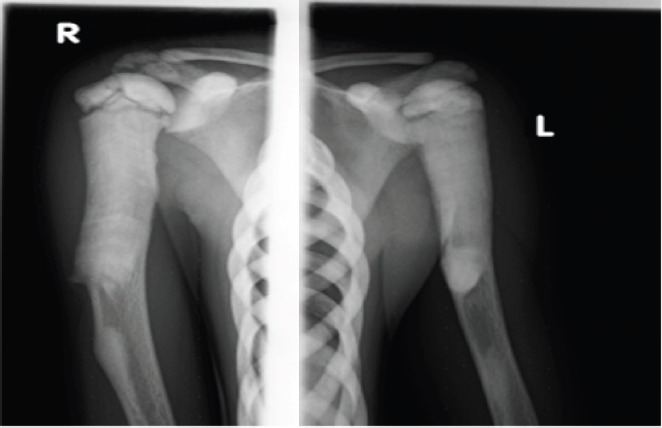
Radiograph of bilateral shoulders and upper arms showing increased bone density involving the medulla and cortex of the diaphysis, metaphysis, and epiphysis with loss of cortical and medullary differentiation
